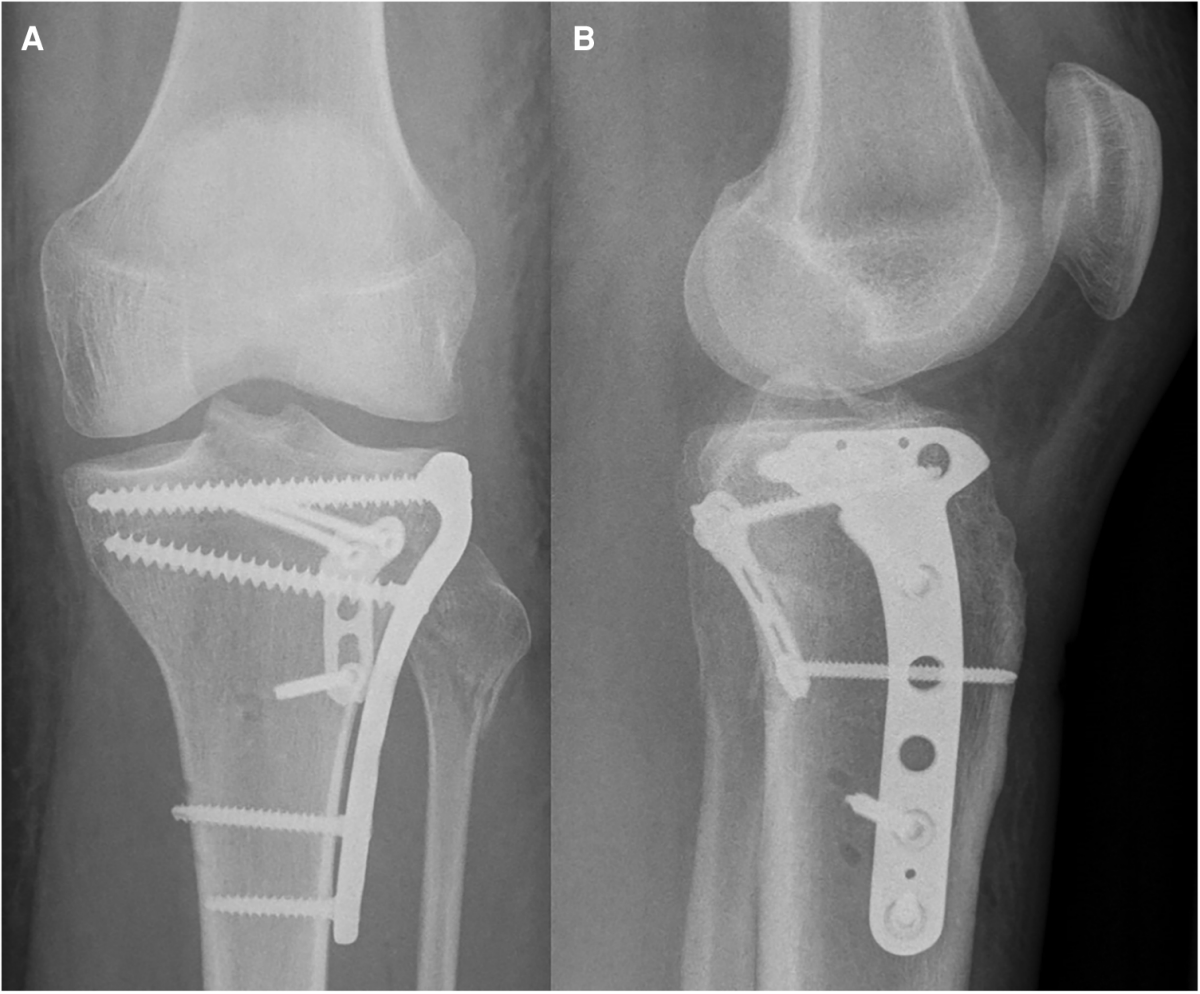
Case 4, posterolateral split impression fracture, postoperative AP (A) and lateral (B) x-ray after ORIF with two angular stable plates using the frosch approach.
